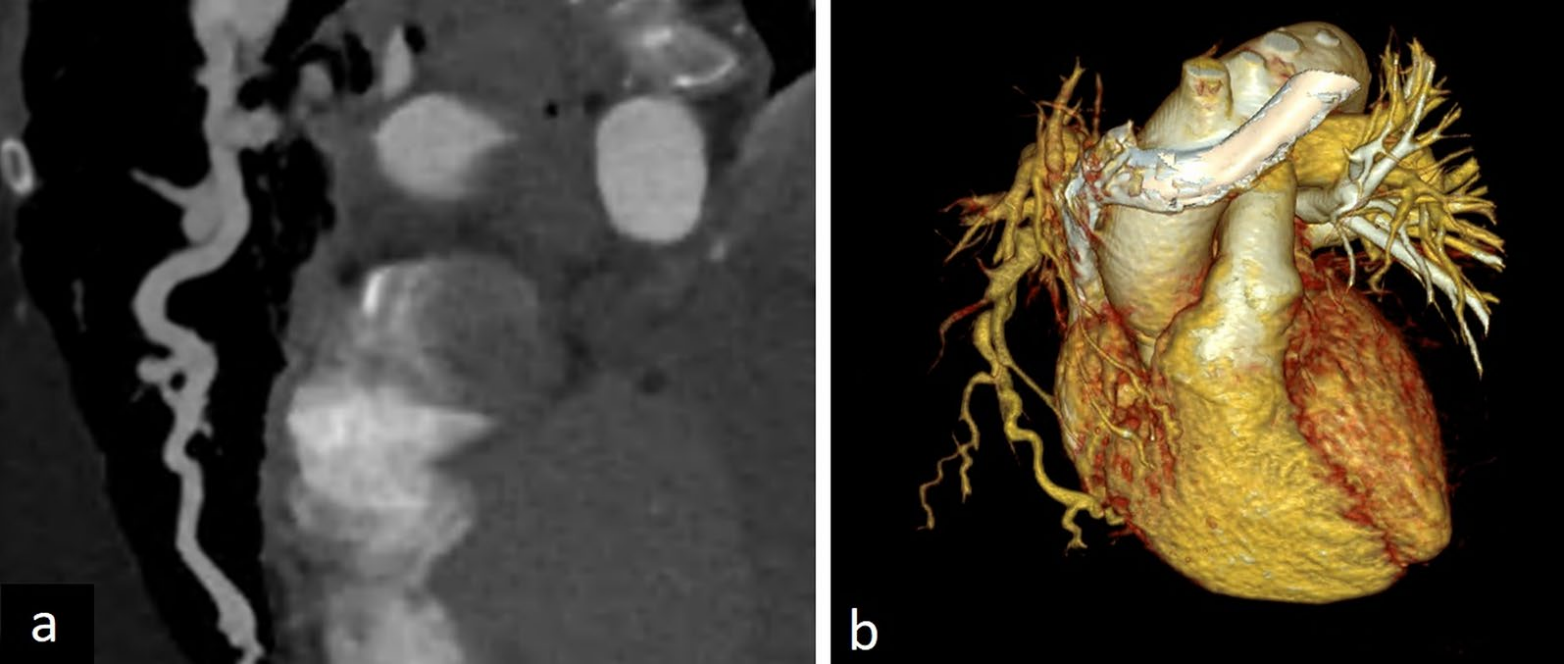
Pulmonary varix. Curved multiplanar reconstruction (a) and 3D volume-rendered CT image (b) demonstrate pulmonary varix in a 73-year-old female
